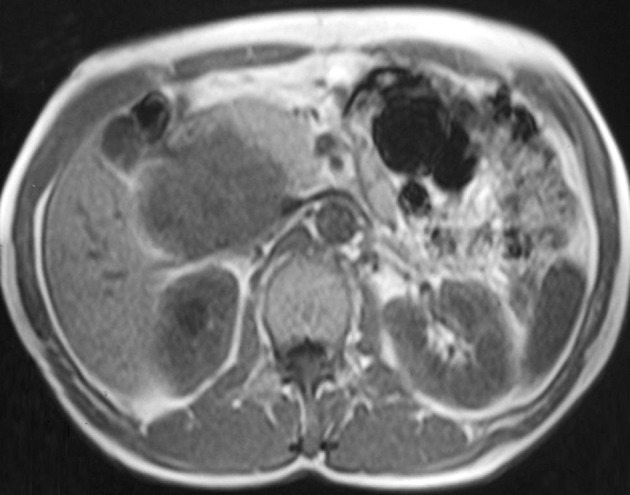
Axial T1-weighted MR image shows isointensity of the mass compared to the skeletal muscle.
